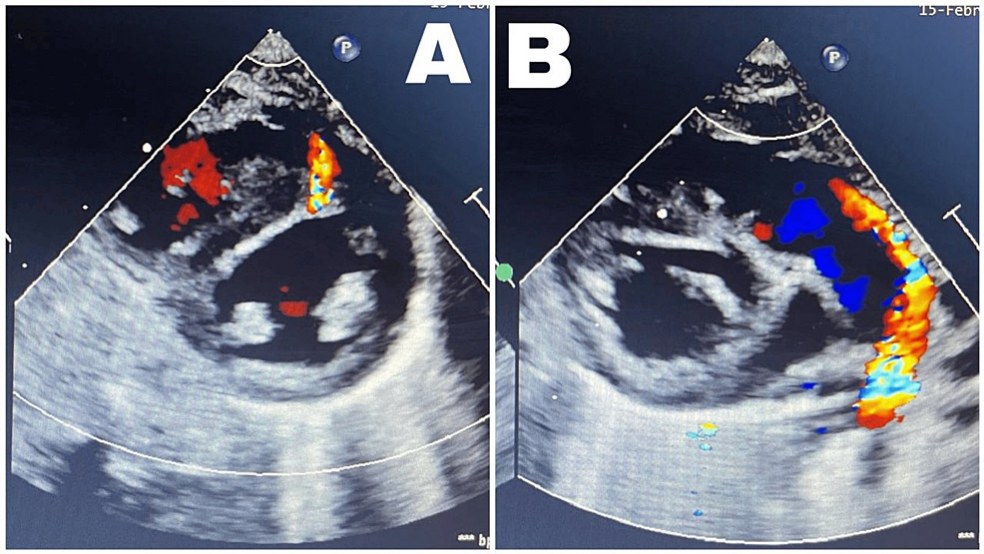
Echocardiography An echocardiography study revealed a small anterior muscular ventricular septal defect (VSD) (A) and moderate patent ductus arteriosus (PDA) (B).
